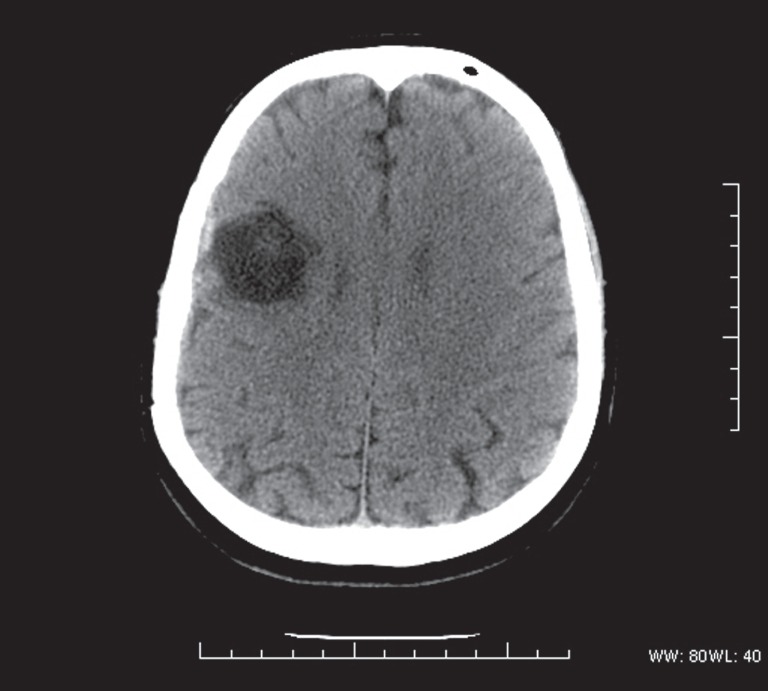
Brain CT showing cerebral abscess of the front-parietal region.
